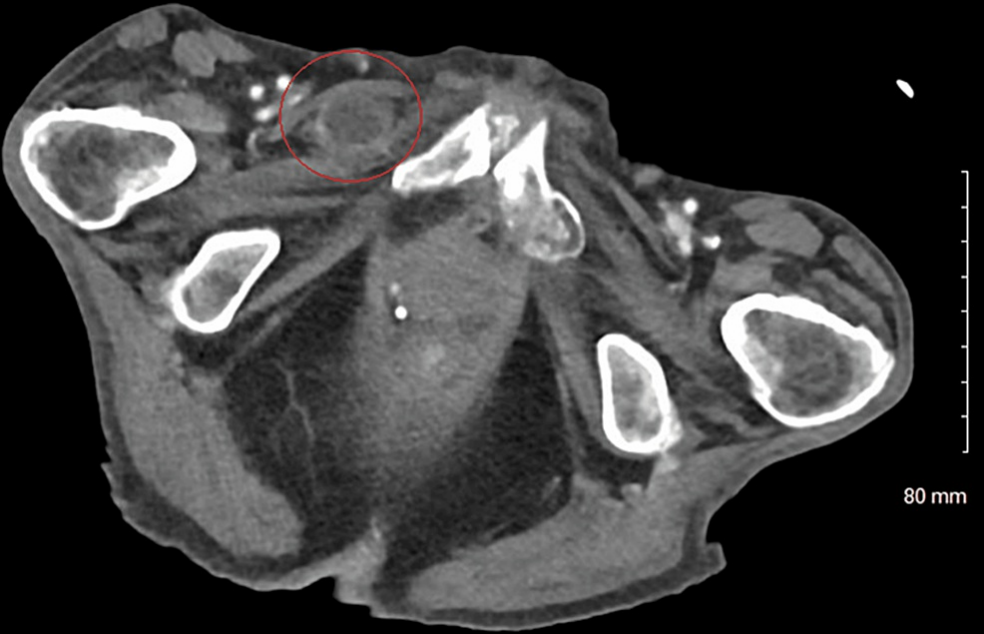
Axial computed tomography of our patient in 2022 demonstrating a recurrent right obturator hernia containing a piece of incarcerated small bowel (red circle).
